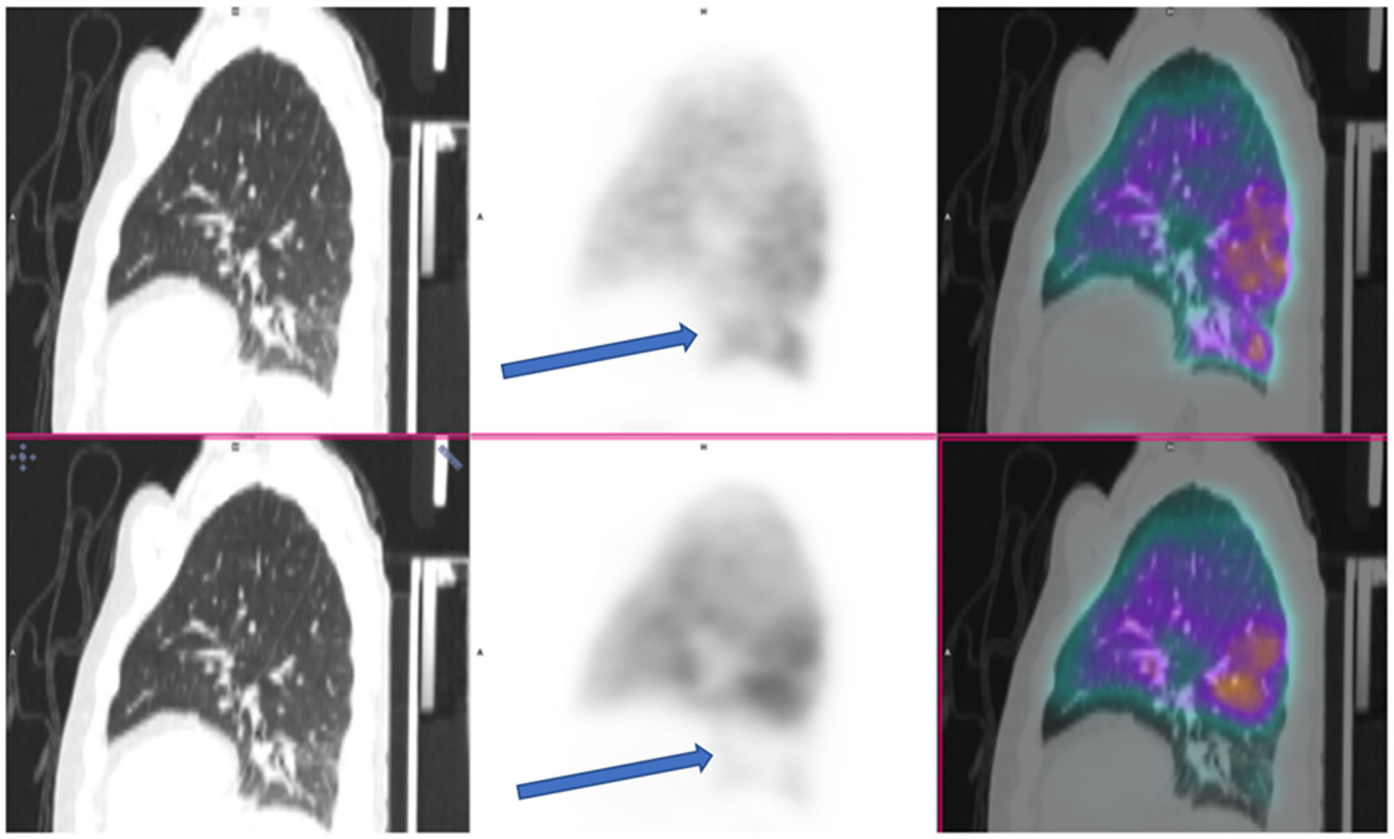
V/Q PET-CT, ventilation on the top and perfusion on the bottom, described as positive by one nuclear physician out of four with a mismatched perfusion defect on lower lobe (arrows), excluded by the three others because of a linear pattern of the defect and not wedge shape.
